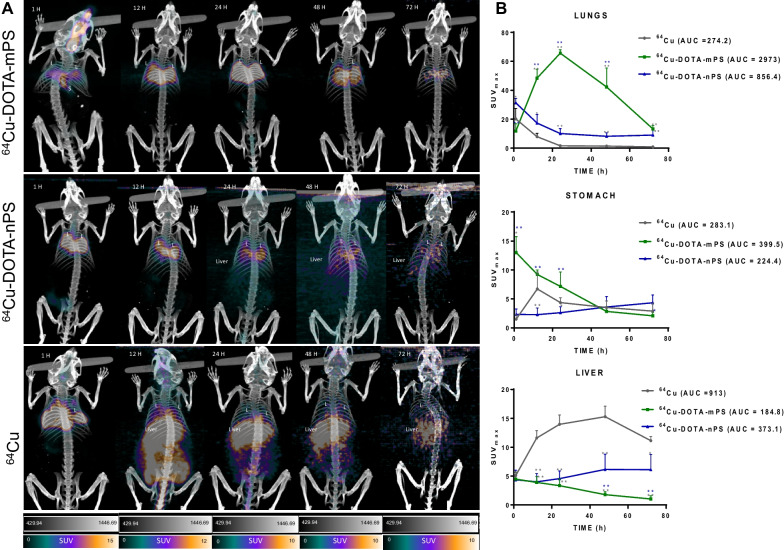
(A) Representative PET-CT images of intra-tracheally instilled ^64^Cu-DOTA-mPS, ^64^Cu-DOTA-nPS and ^64^Cu at all time points displaying retention of polystyrene in lungs compared to ^64^Cu that is rapidly cleared from the lungs post instillation. (B) The graphs represent SUV_max_ for important organs such as lungs, stomach and liver along with the AUC which is maximum for mPS in lungs and ^64^Cu in liver. AUC = area under curve; mPS = micro-polystyrene; nPS = nano-polystyrene; L = lungs; S = stomach (P* < 0.05, *P* < 0.005, * significance in comparison to ^64^Cu; * significance in comparison to.^64^Cu-DOTA-nPS)
